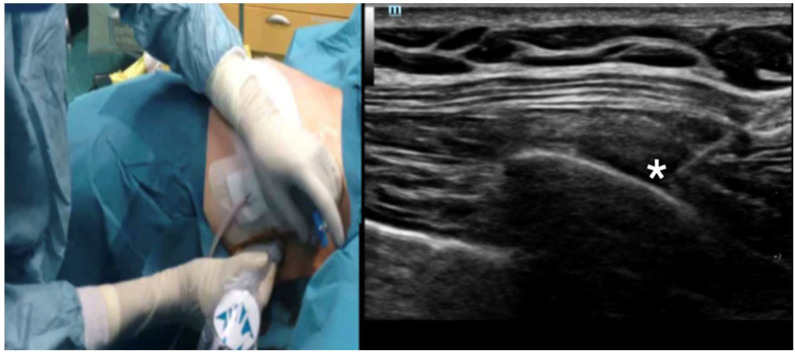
Technique for positioning of the catheter for continuous analgesia in Serratus Anterior Plane Block. Left panel: catheter placement via a sterile technique. Right panel: ultrasound in-plane view of the maneuver. The needle tip was positioned between the Serratus muscle and the surface of the fifth rib in a craniocaudal direction, using the “deep technique”. The * marks the needle tip in the fascial plane between the rib and the Serratus muscle.
